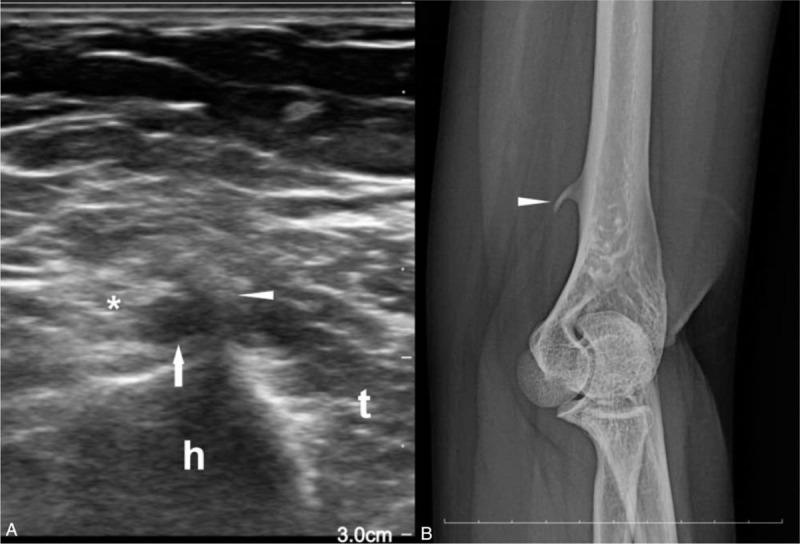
Image of the left distal humerus. A) Ultrasonography. Curved echogenic structure (arrowhead) projecting from the humerus (h) is observed superficial to the median nerve (asterisk) and brachial artery (arrow). t; triceps. B) Simple radiograph. Medial oblique view of the left elbow showed supracondylar process (arrowhead) 4.2 cm proximal to the medial epicondyle.
